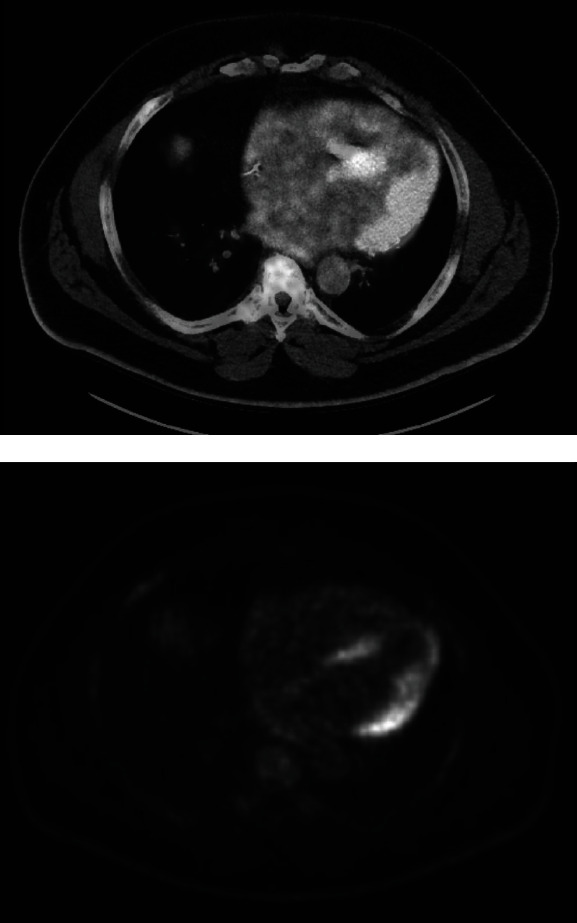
Active sarcoid seen in myocardium on FDG PET CT. FDG PET CT showing abnormal uptake in the myocardium as well as apical inferior wall with sparing of the apex with no extracardiac FDR uptake.
